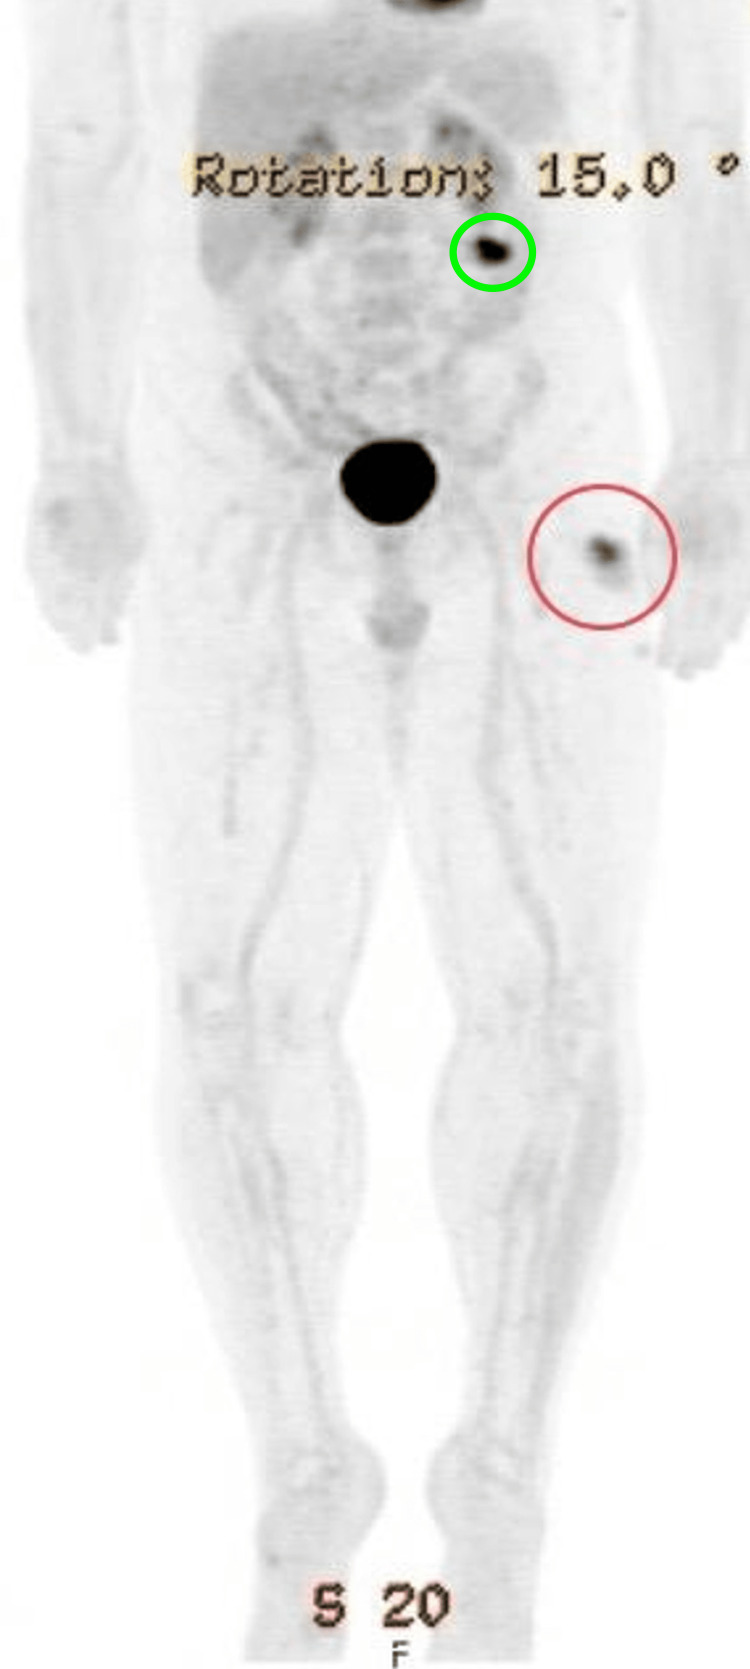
PET scan showing mild fluorodeoxyglucose (FDG) - avid activity in the left upper thigh (red circle) and mild fluorodeoxyglucose (FDG) - avid activity in the left anterior abdominal wall (green circle)
